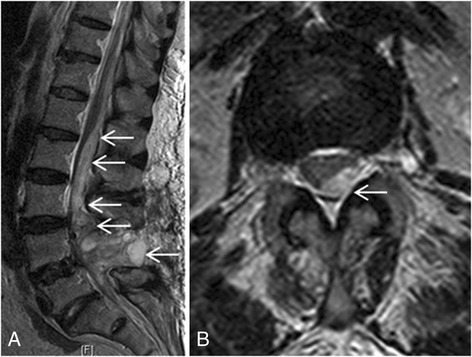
Magnetic resonance images of epidural hematoma and cauda equina syndrome. This patient had progressive peri-anal numbness and left lower extremity weakness on postoperative day 1. a Postoperative sagittal magnetic resonance imaging revealed epidural hematoma extending from L4–5 to the L2 level (arrows). b Cross-sectional magnetic resonance imaging revealed epidural hematoma at L2–3 (arrow) with compression-induced cauda equina syndrome
